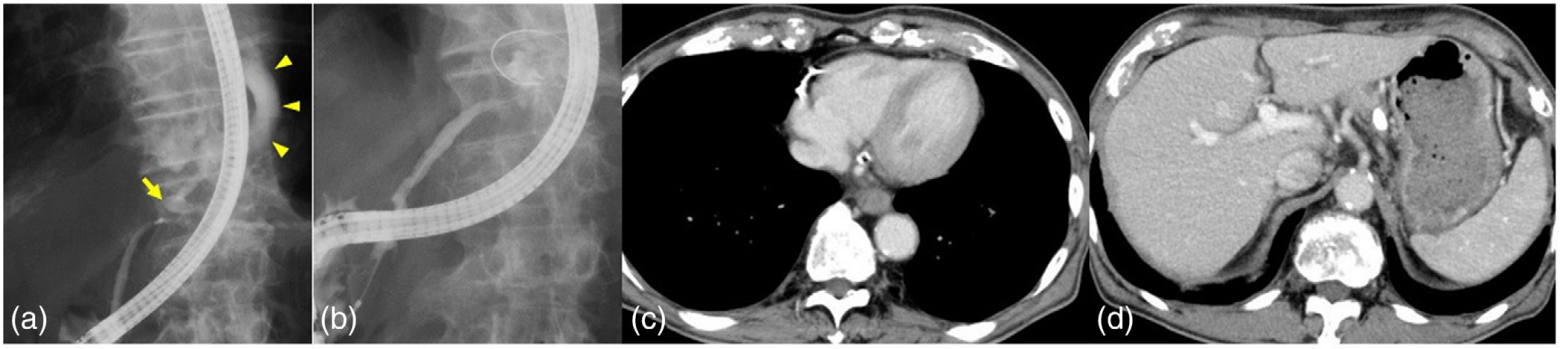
Endoscopic retrograde pancreatography: (a) Leakage of contrast medium was observed from the vicinity of the pancreatic stone to the outside of the pancreas, and a pancreatic pseudocyst and mediastinal pseudocyst were visualized (yellow arrowhead: pseudocyst, yellow arrow: pancreatic stone). (b) A guidewire was inserted into the pancreatic pseudocyst. (c, d) Follow‐up computed tomography after endoscopic transpapillary drainage. The (c) mediastinal pseudocyst and (d) pancreatic pseudocyst had completely disappeared
